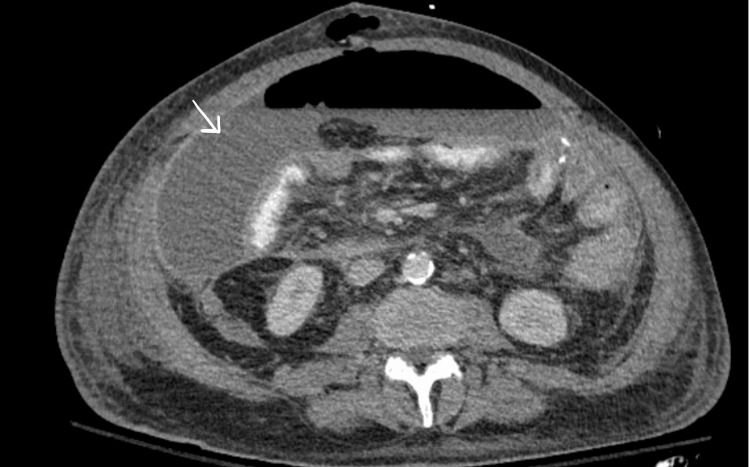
CT abdomen and pelvis with contrast - image 4 The white arrow indicates fluid accumulation. There is also associated intra-abdominal free air consistent with an intra-abdominal abscess CT: computed tomography
